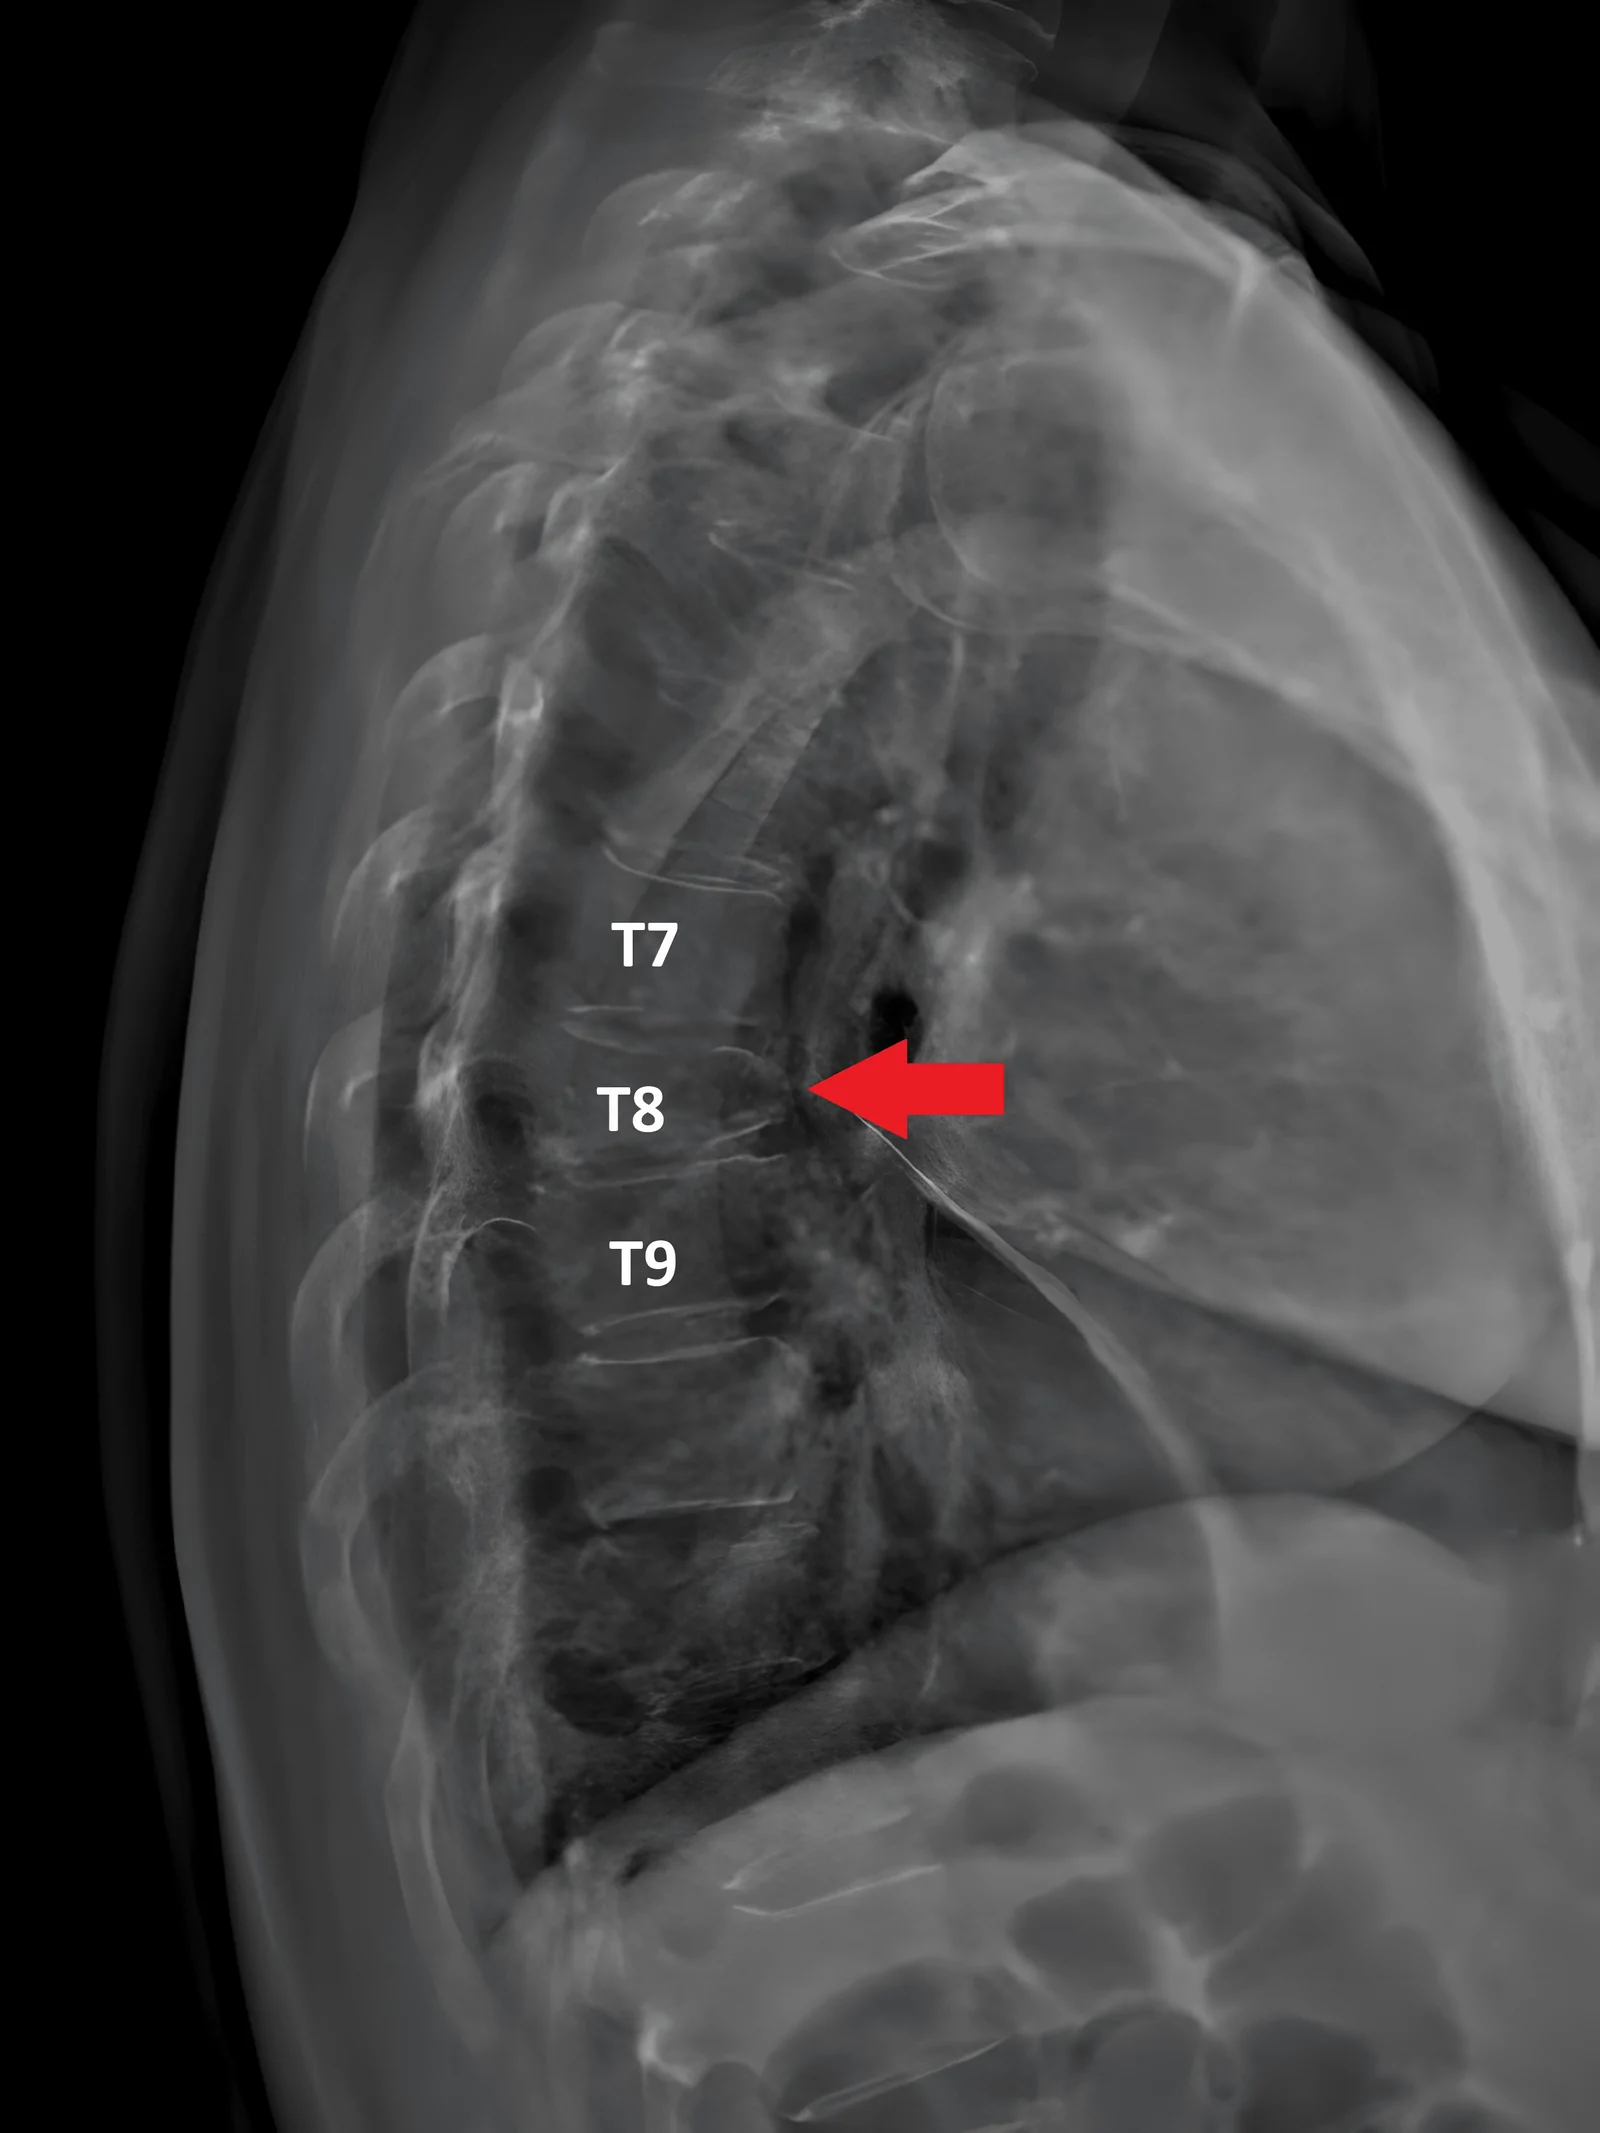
Radiograph of the thoracic spine. Osteopenia with mild osteoporotic fractures is identified at the mid thoracic spine (red arrow).
